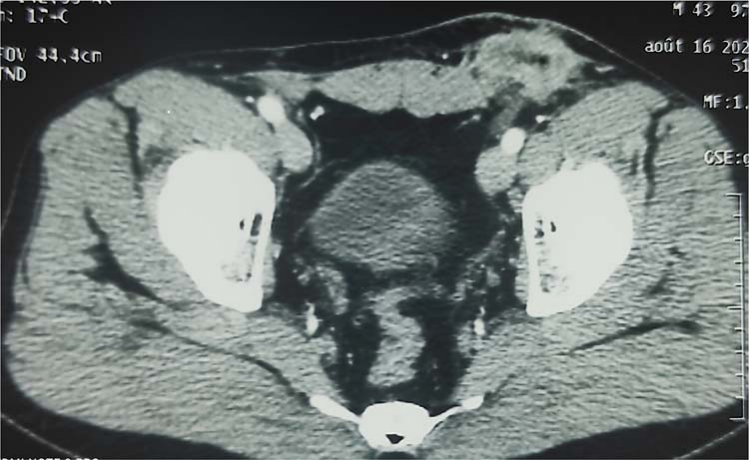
Enhanced CT scan showing well-limited mass in the left inguinal region with heterogeneous enhancement and central hypodensity related to the infected mesh.
